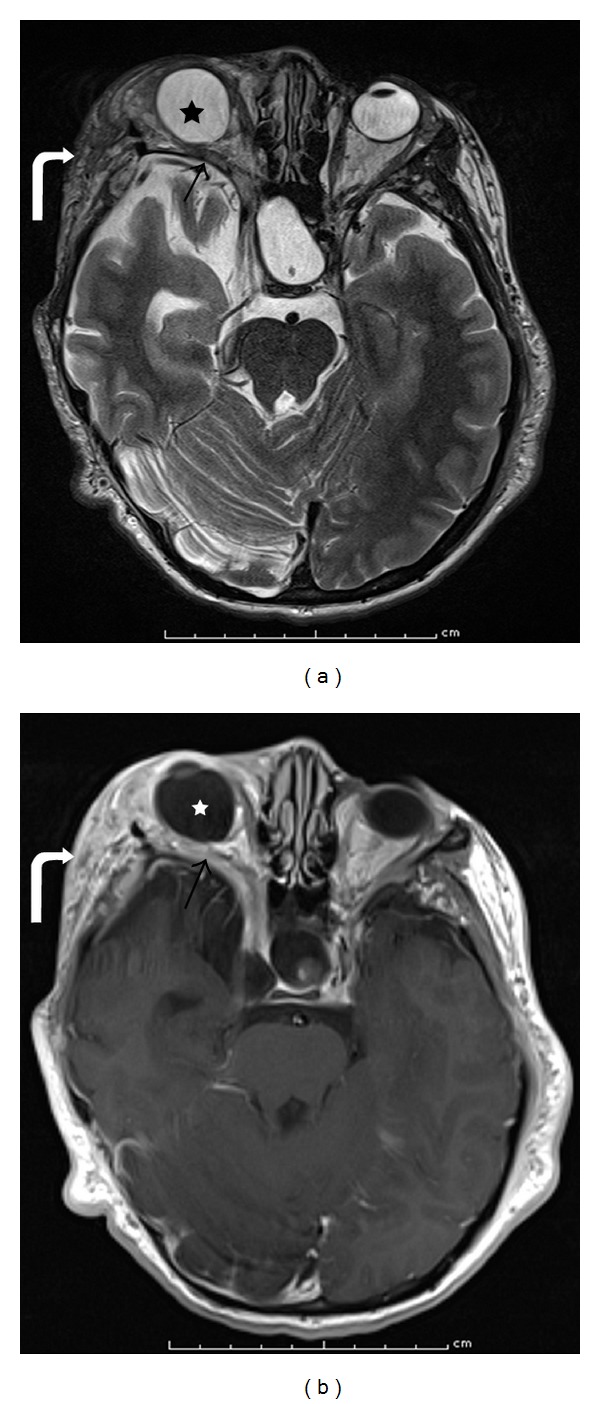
11 years old with right pulsatile proptosis. MR axial T2-weighted image (a) and T1-weighted postcontrast image (b) showing sphenoid wing dysplasia with mild temporal lobe herniation (arrow); plexiform neurofibroma with areas of T2 hyperintensity, heterogeneous enhancement, and extension lateral to the orbit into the infratemporal fossa (curved arrow); and buphthalmos and exophthalmos (star) of the right orbit.
